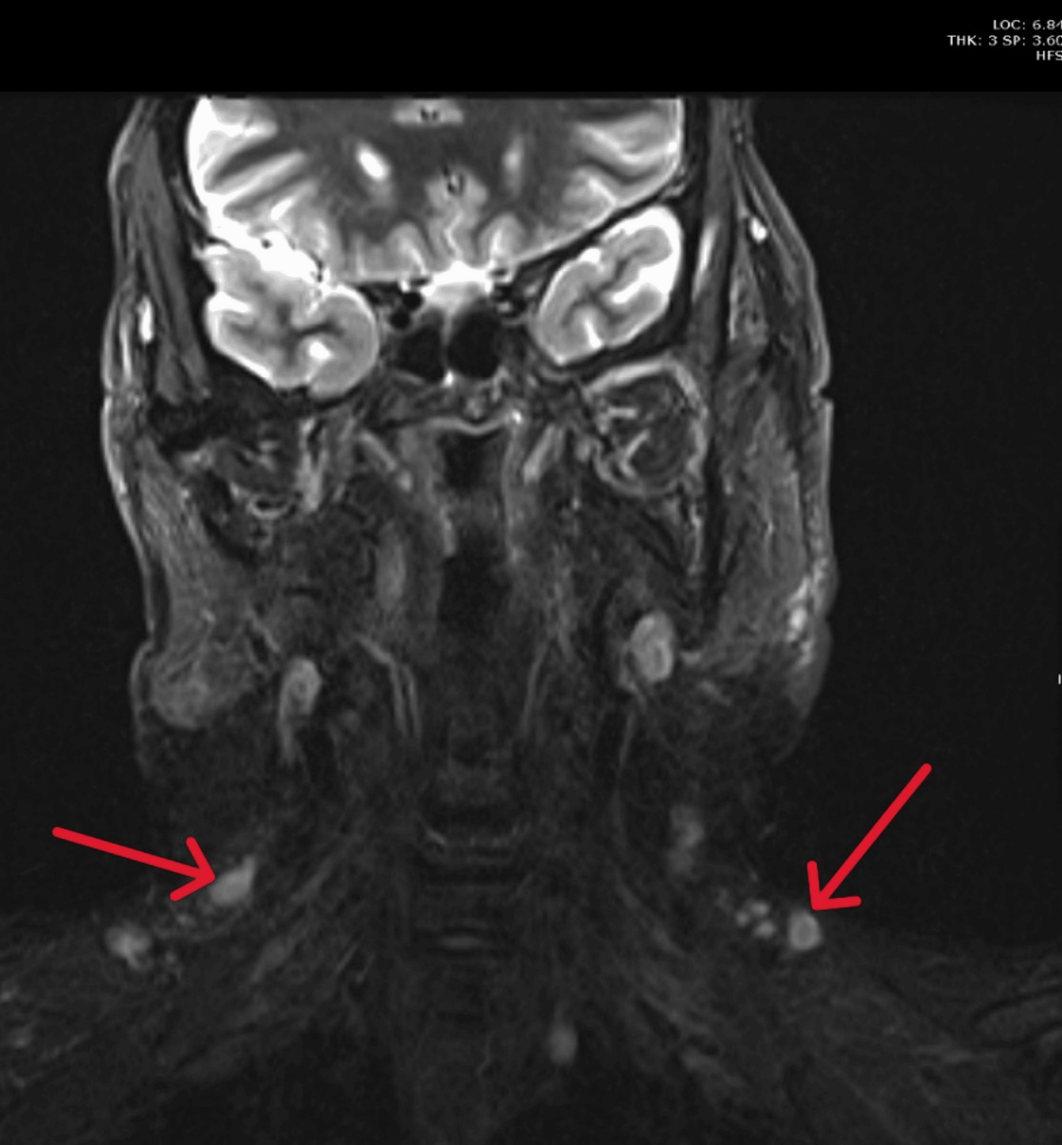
MRI of the neck revealed multilevel bilateral prominent lymph nodes, seen mainly within the supraclavicular regions bilaterally (red arrows).
